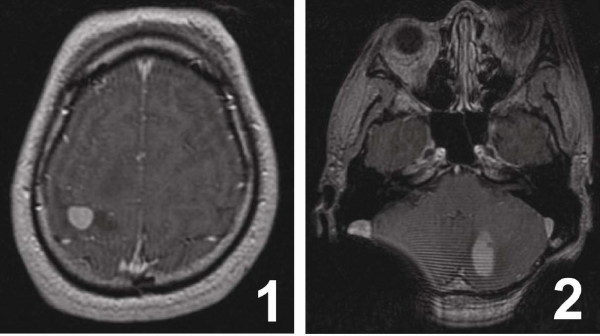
MR imaging of the head in 2009.(1) The MRI T1 Weighted Imaging shows that there are multiple masses with high signals in the margin and low signals in the center, and T2 Weighted Imaging shows equal signals, in the right cerebral hemisphere, especially in the right frontal-parietal lobe and the basal ganglia area, and edema with low signals could be seen around the mass. (2) The left cerebellum is also visible of abnormal signals.
